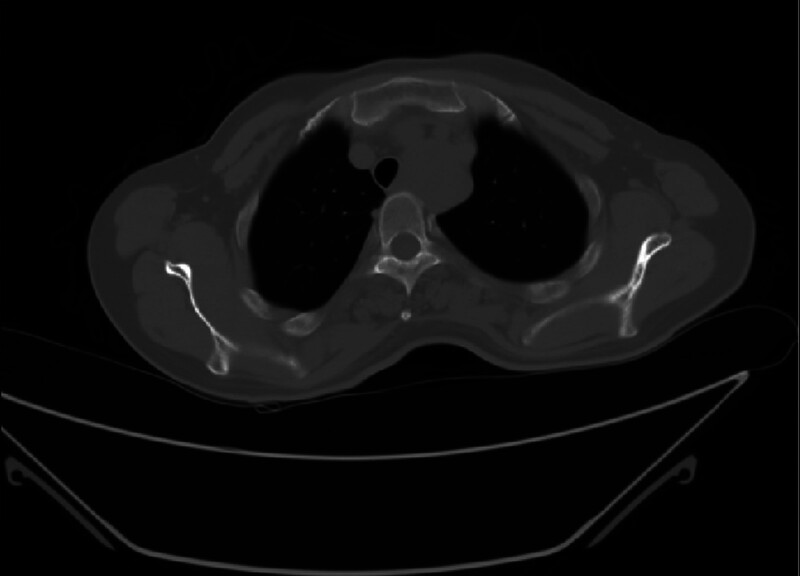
Chest computed tomography (CT) showed a mass-like soft tissue shadow in the upper esophagus measuring approximately 39 mm × 34 mm.
